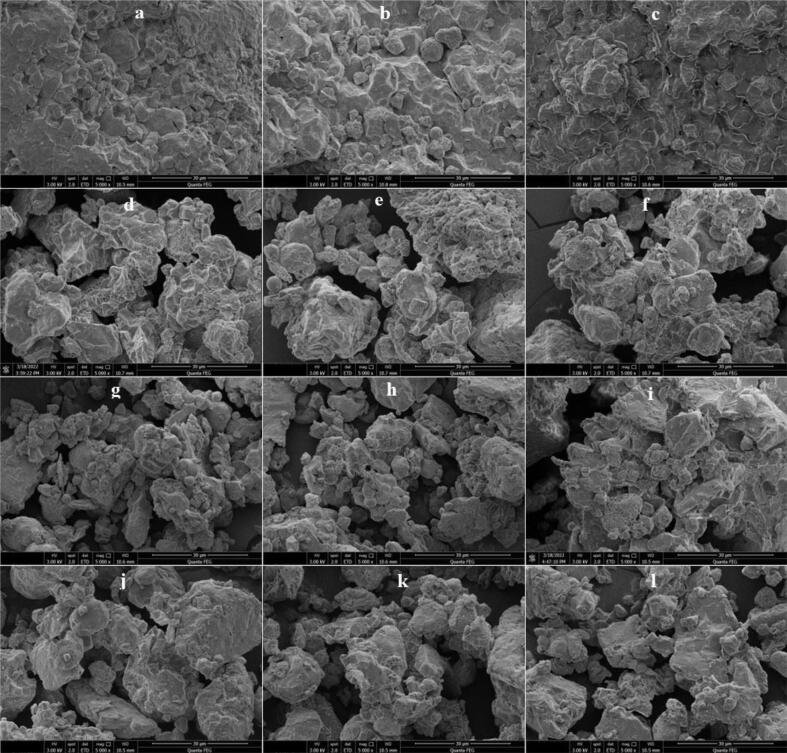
Effect of ultrasound and drying temperatures on the microstructure of SeGBR.
